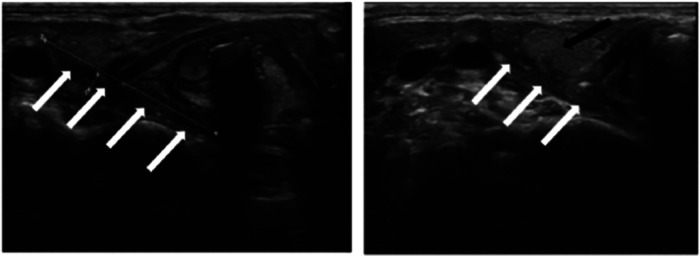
Ultrasound of neck. Tubular hypoechoic fistula into the posterior part of the right lobe of the thyroid gland. White arrow: fistula; Black arrow: thyroid gland.
